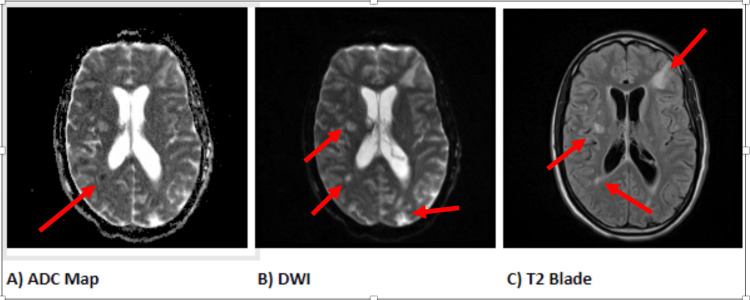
MRI images demonstrating multiple T2 hyperintense foci in deep white matter (A-C), some of which show diffusion restriction with low ADC (A), indicated by arrows ADC, apparent diffusion coefficient
